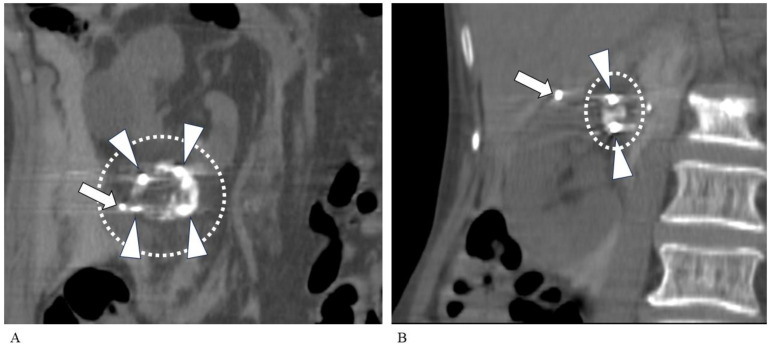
Differences in operative time and number of needles needed for tumors of different sizes. Coronal CT images taken 15 min after the second ablation. (A) An 86-year-old man with a left RCC visualized by iodized oil. The lesion is completely covered by a large ice ball (dashed oval). The tumor diameter was 34 mm, requiring 4 cryoneedles (arrowheads). The total operative time was 230 min. Arrow: needle for hydrodissection. (B) An 86-year-old woman with right-sided RCC. The tumor diameter was 13 mm, requiring 2 cryoneedles (arrowheads). A small ice ball is seen (dashed oval). The total operative time was 125 min. Arrow: needle for hydrodissection. CT, computed tomography; RCC, renal cell carcinoma.
